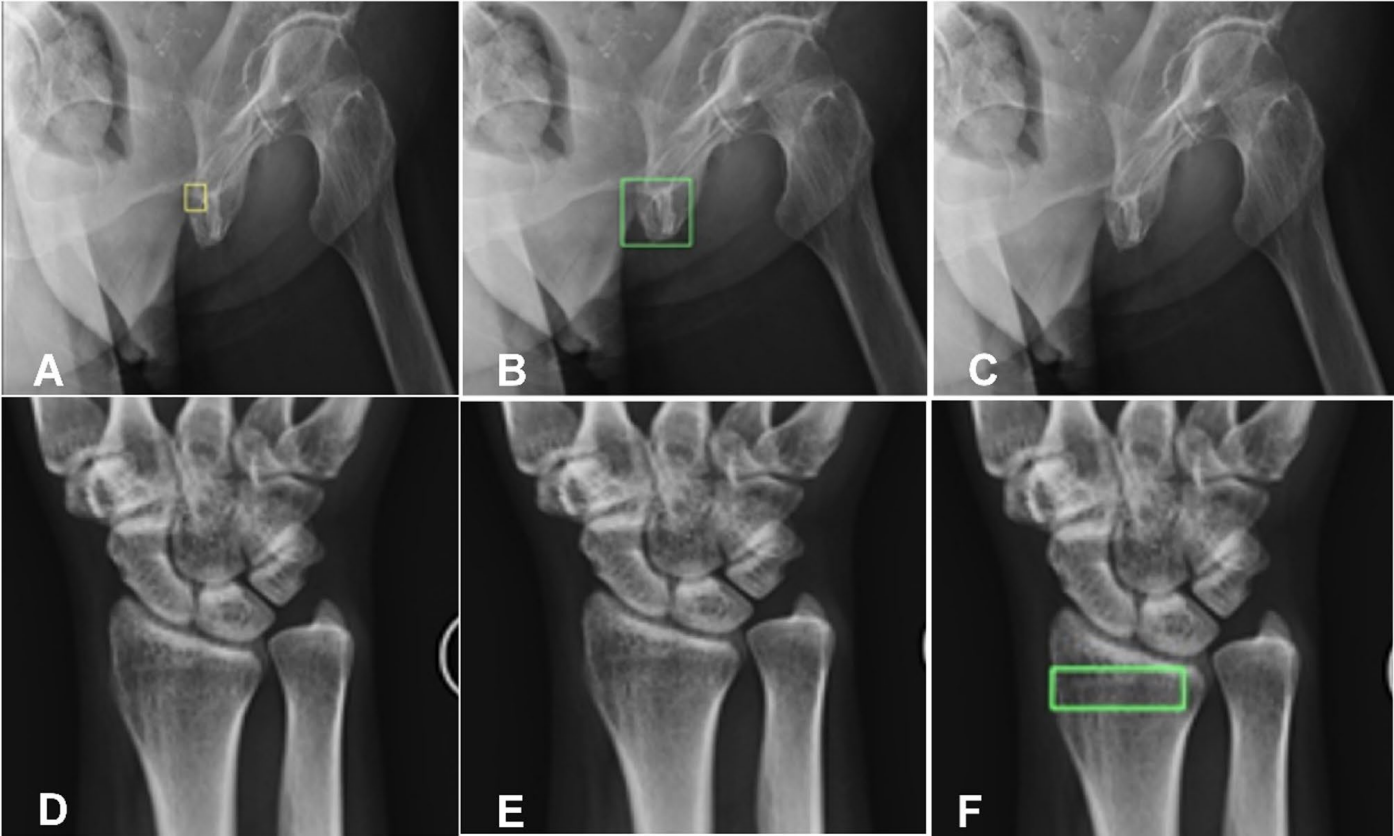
Effects of the FAMO (Feature Ambiguity Mitigate Operator) plus ResNeXt101+FPN model and the ResNeXt101+FPN model only, with the FAMO plus ResNeXt101+FPN model being helpful in finding bone fractures. (A) A minor fracture was marked by the radiologist (small box) on the radiograph. (B) The fracture line was successfully recognized by the FAMO plus ResNeXt101+FPN model (green box). (C) The ResNeXt101+FPN model did not recognize the fracture. No fracture line was detected on the radiograph by the radiologist (D) or by the FAMO plus ResNeXt101+FPN model (E). But the ResNeXt101+FPN model (causing feature distortion) detected a false positive fracture line (F, green box).
